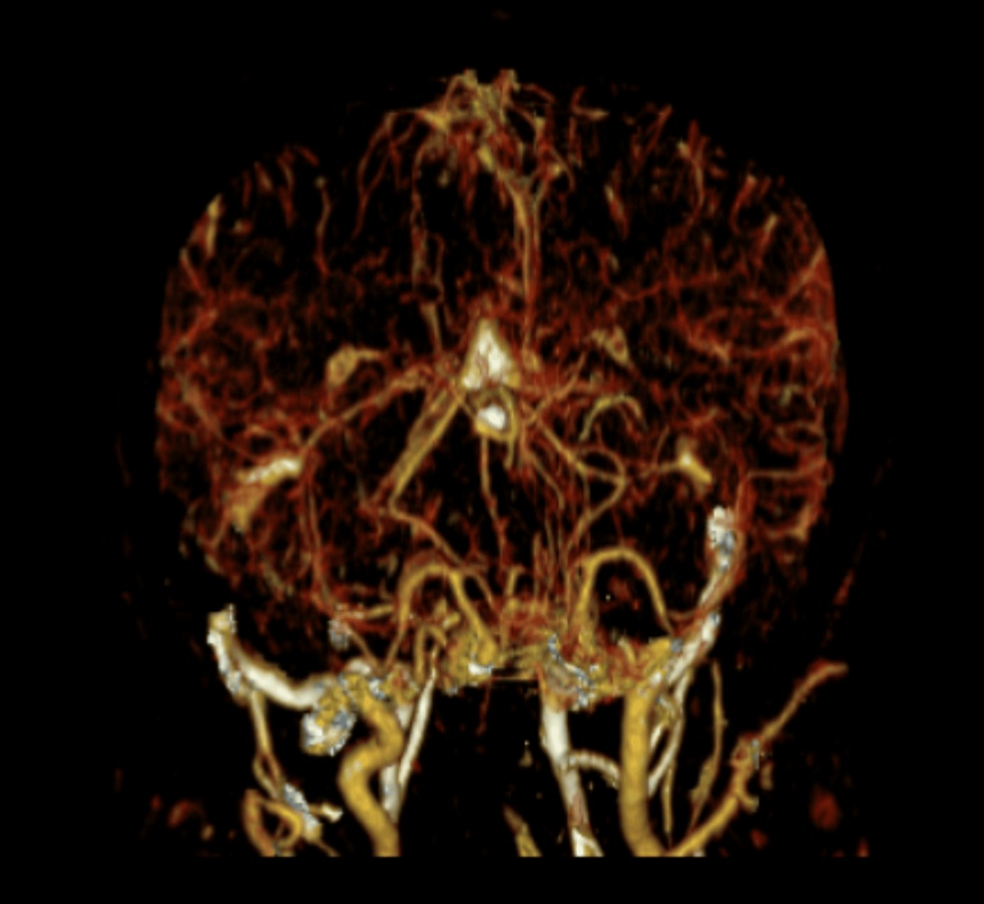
Head CT Venogram CT venogram of the head showing no evidence of dural venous sinus thrombosis.
